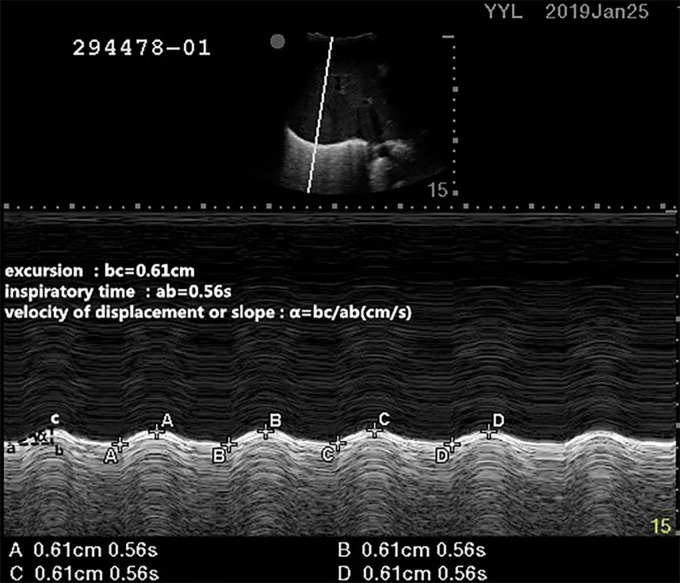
Measurements of the diaphragmatic excursion (DE) and systolic velocity by the ultrasound. During inspiration, the diaphragm contracts and moves toward the probe. During expiration, the diaphragm rebounds and moves away from the probe. The distance between them on the vertical axis during a respiratory cycle was recorded as the diaphragmatic excursion. For systolic velocity, it was equivalent to DE/time of inspiratory time.
